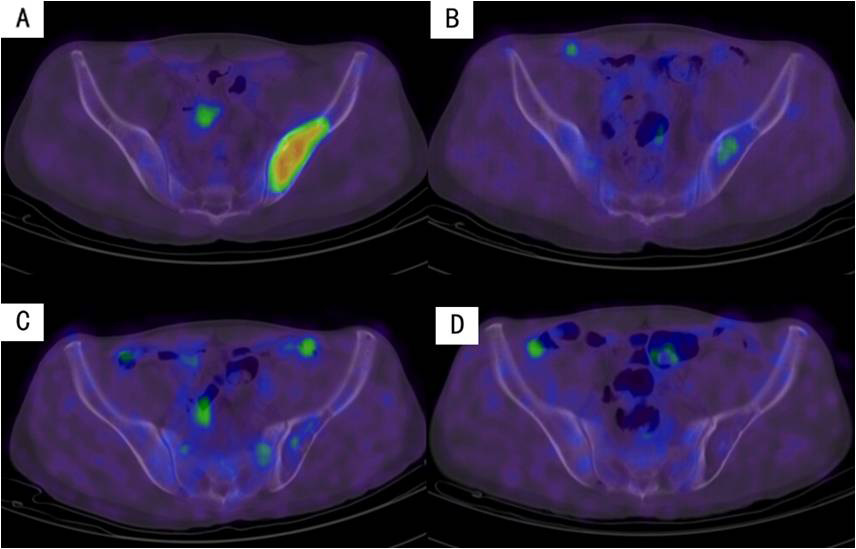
Decreased uptake of ^18^F-FDG in the iliac bone with continued treatment in an MM patient A. On diagnosis. B. After PAD regimen. C. Six months after transplantation. D. Twelve months after transplantation.
